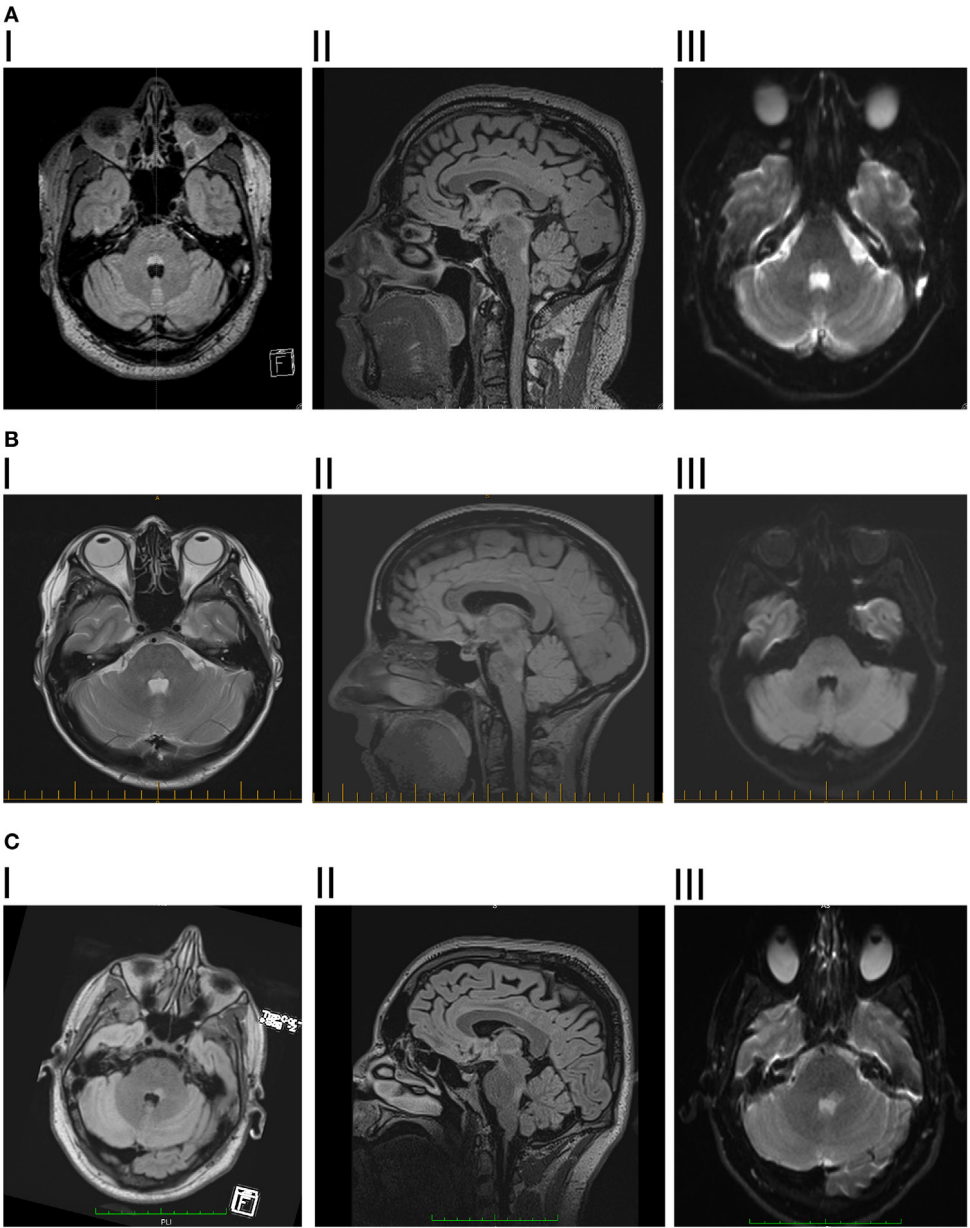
(A) I. Patient 1, axial fluid-attenuated inversion recovery (FLAIR) showing hyperintense lesion in posterior pontine tegmentum. II. Saggital FLAIR showing vertical extent of the lesion. III. Diffusion-weighted imaging showing mild diffusion restriction. (B) I. Patient 2, axial dual showing hyperintense lesion in posterior pontine tegmentum. II. Saggital FLAIR showing vertical extent of the lesion. III. Diffusion-weighted imaging showing mild diffusion restriction. (C) I. Patient 3, axial flair showing hyperintense lesion in posterior pontine tegmentum, slightly more significant on the right side. II. Saggital FLAIR showing vertical extent of the lesion, slightly larger than the previous two patients, consistent with there being some slowing of vertical saccades. III. Diffusion-weighted imaging showing mild diffusion restriction.
